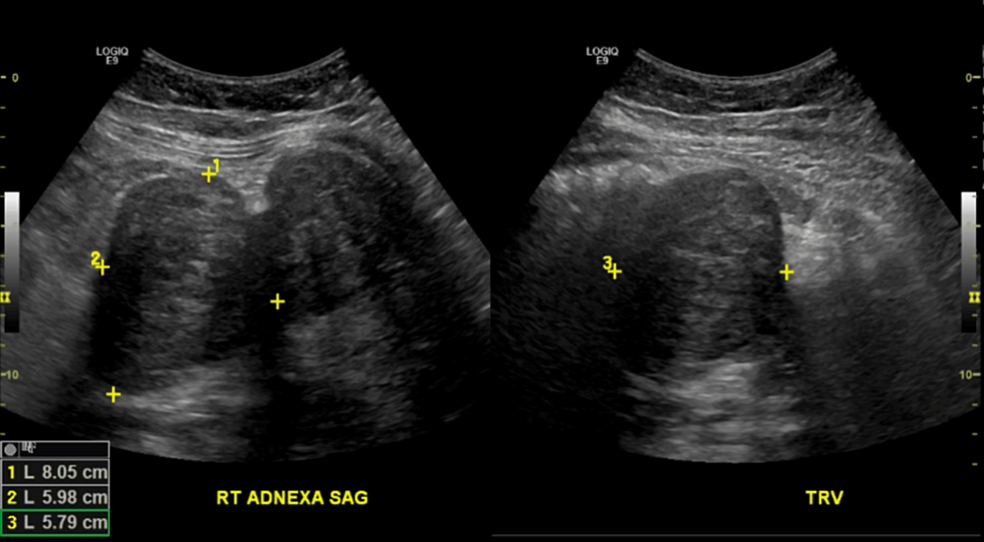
Transabdominal ultrasound
